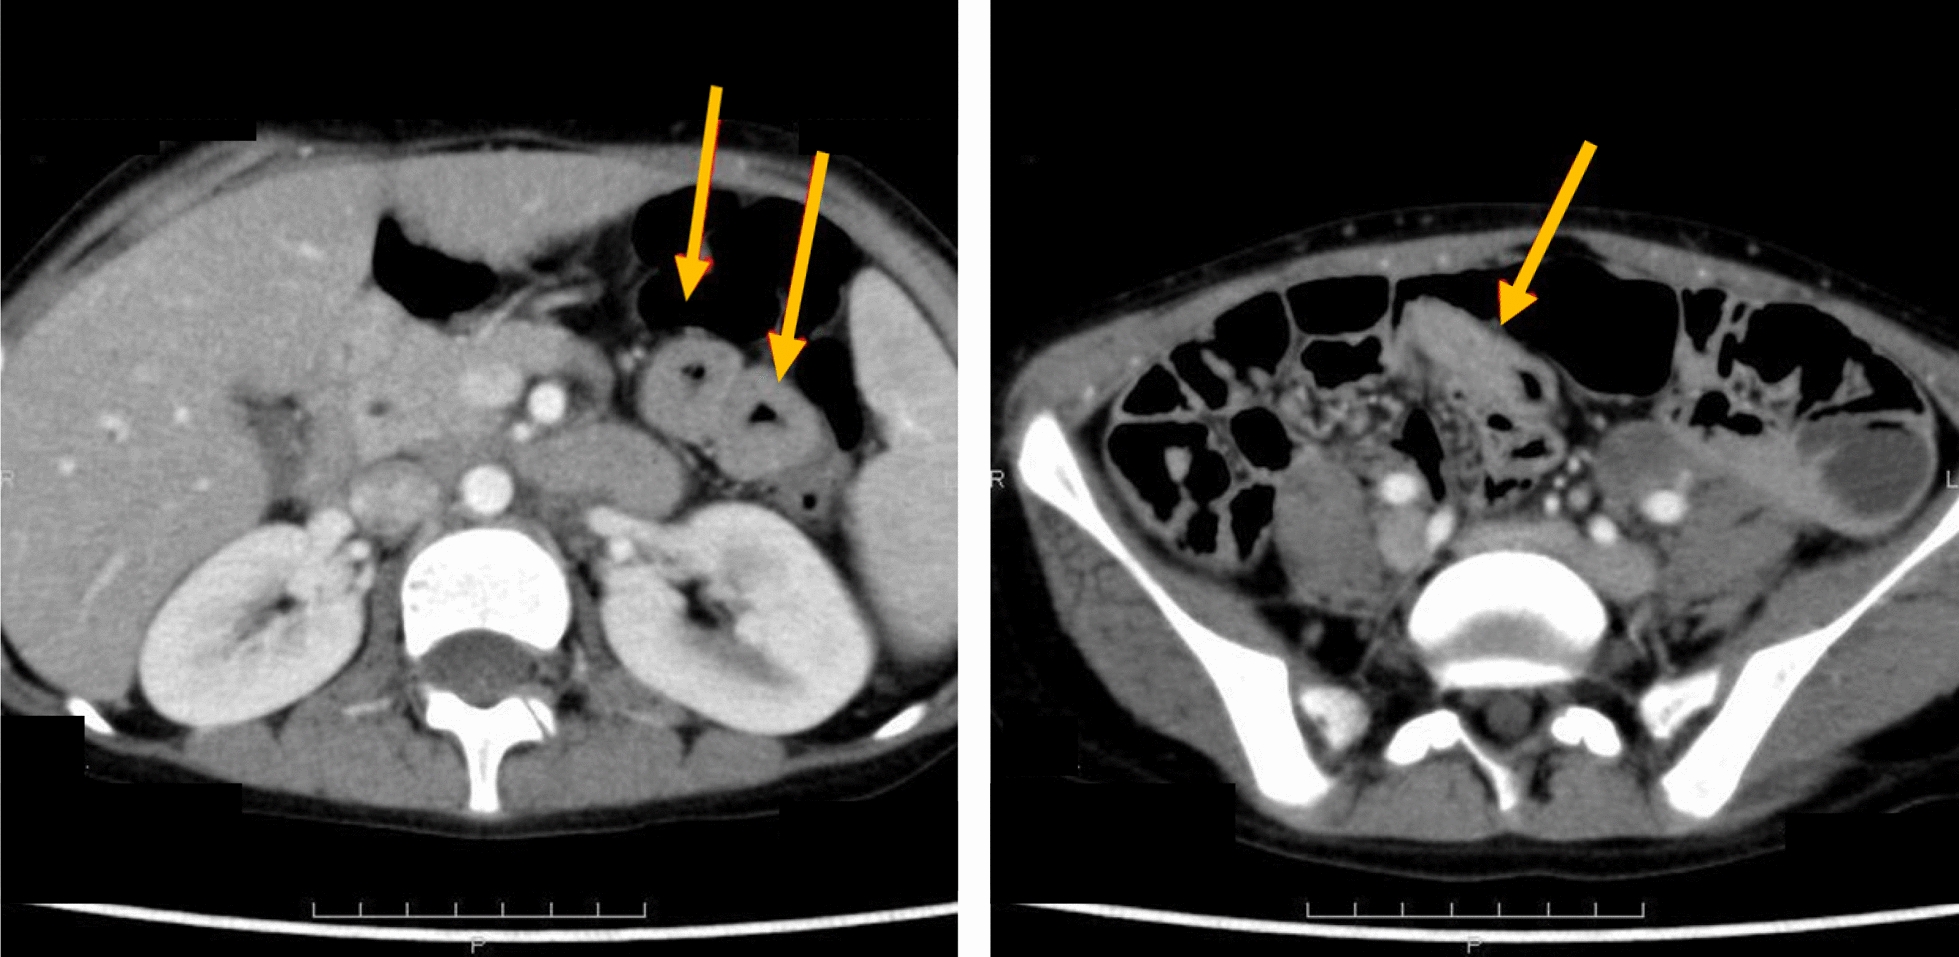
Contrast-enhanced computed tomography of the abdomen: Intestinal wall thickening and lumen narrowing with contrast enhancement, observed from the duodenum to the jejunum, are indicated with arrows
